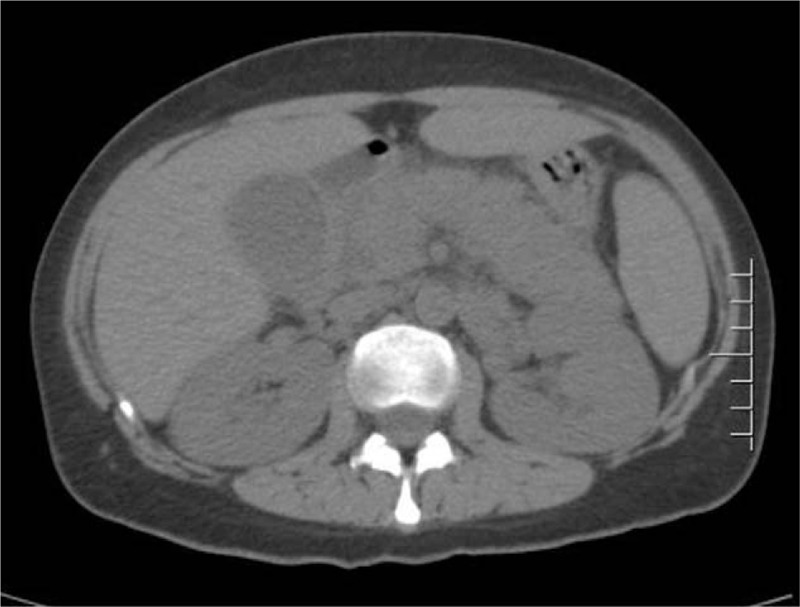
CT of the abdomen revealed a swollen pancreas with blurred edges and thickened left prerenal fascia, which suggested the diagnosis of AP. CT = Computed tomography, AP = Acute pancreatitis.
